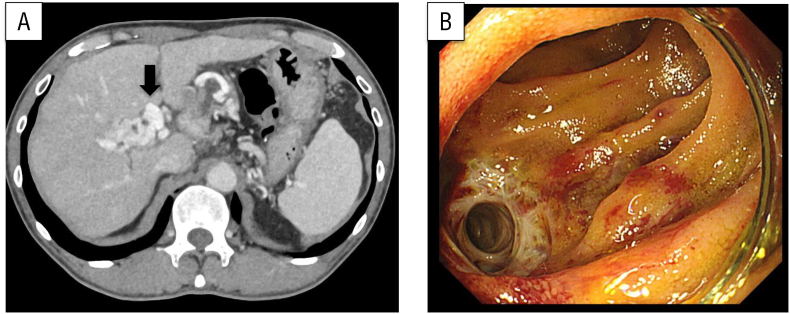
(A) Computed tomography showed ectopic varices around the choledochojejunostomy site (arrow). (B) Enteroscopy revealed ectopic varices around the elevated jejunum at the choledochojejunostomy site.
